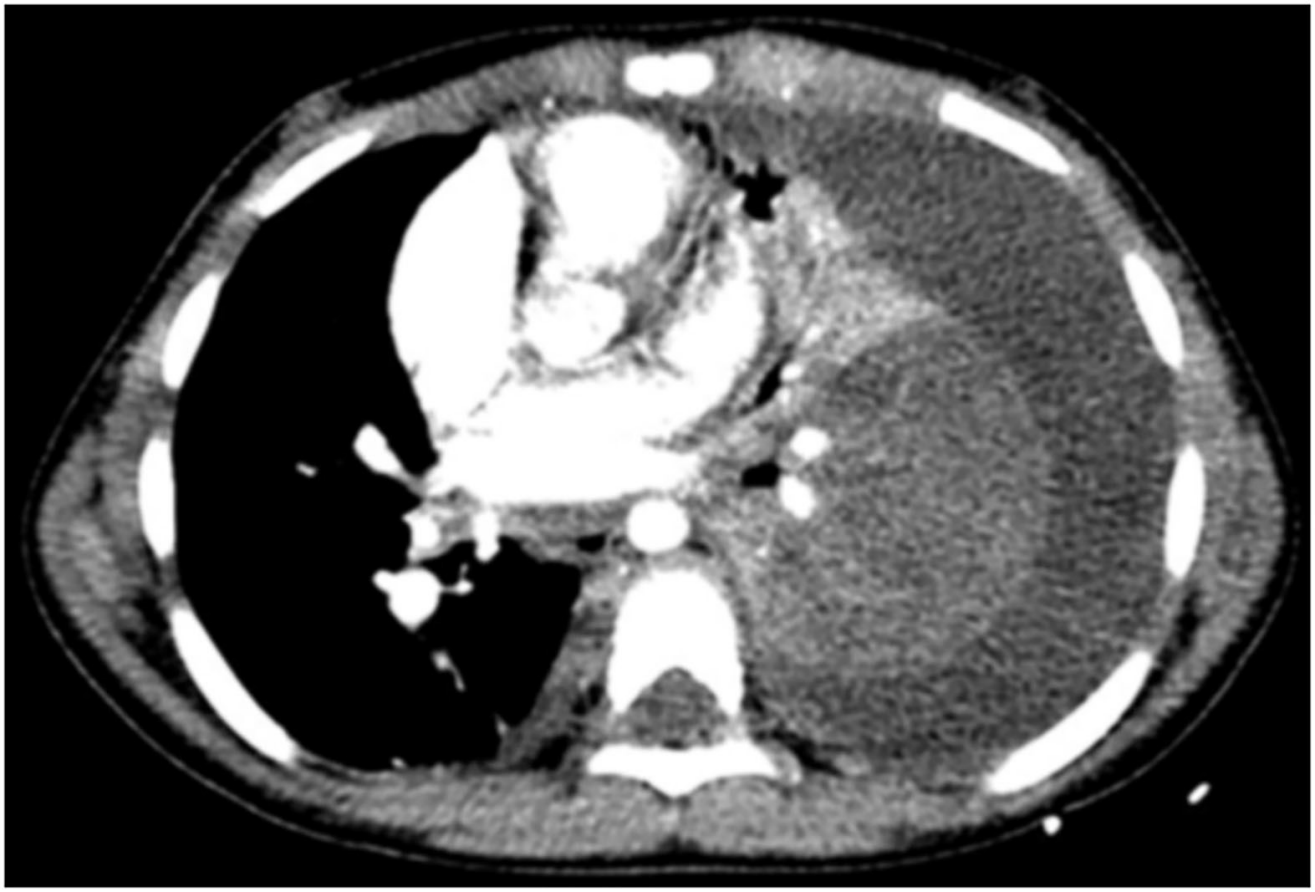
A 5-year-old patient with NP. Transverse slice of chest CT with injection in the mediastinum window shows heterogeneous enhancement of the parenchyma of the left lower lobe in keeping with NP before appearance of cavities. Also visible is a left pleural effusion causing partial atelectasis of the left lung.
